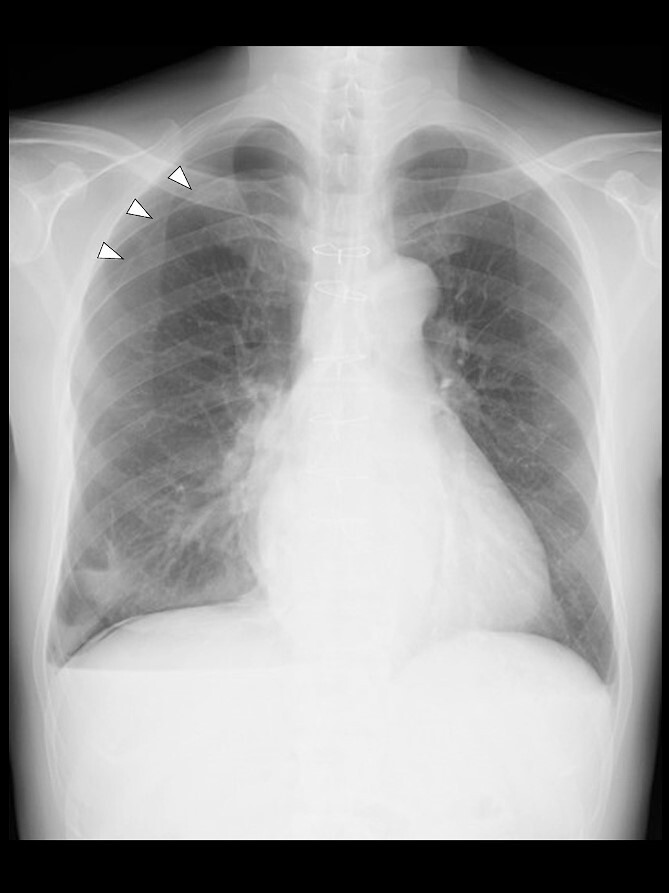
A chest radiograph in the upright position on POD 1 showing a right pneumothorax (arrowheads).
